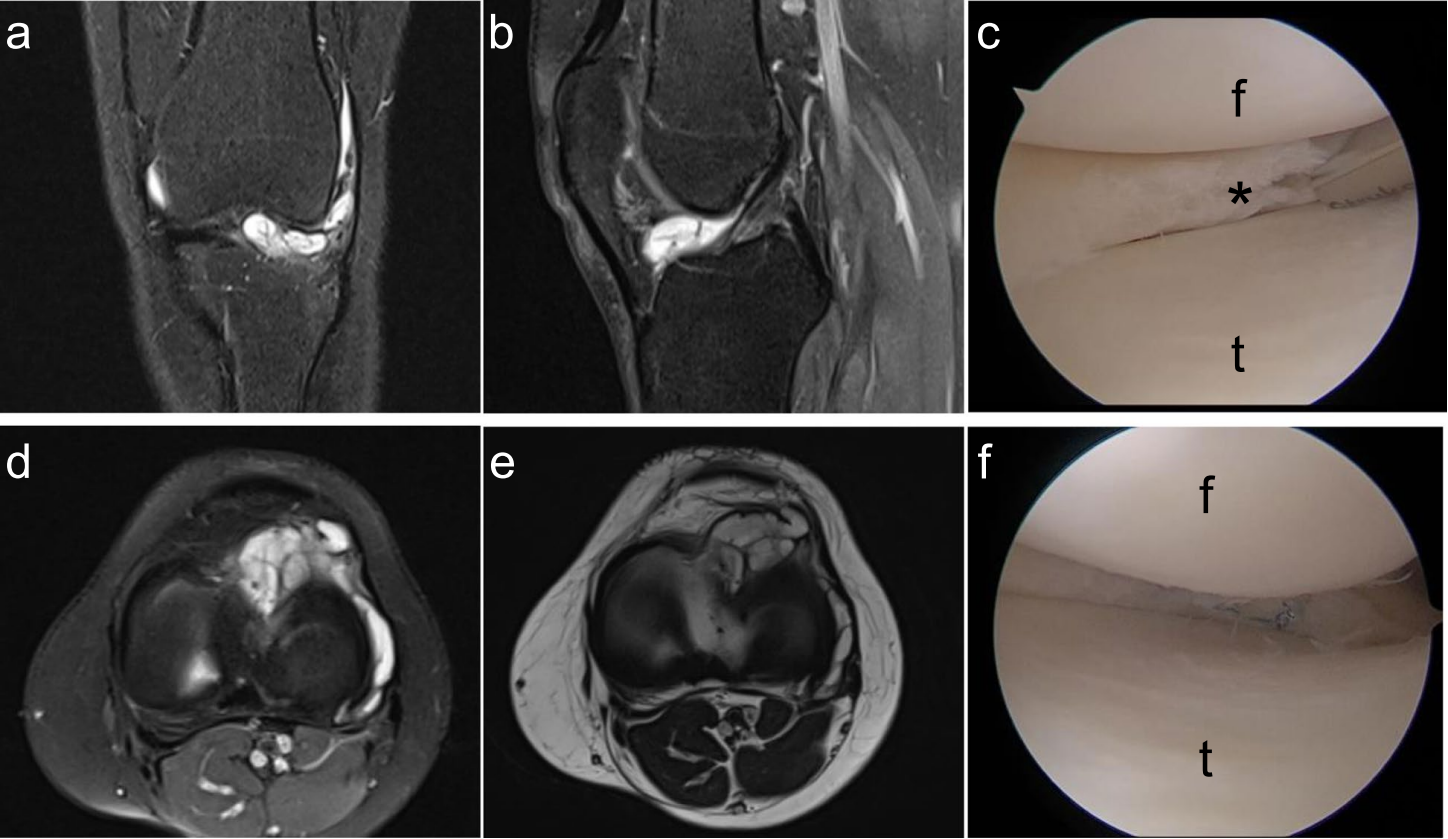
a and b Coronal and sagittal magnetic resonance image (MRI) of left knee with lateral-sided parameniscal cyst. c arthroscopic view of horizontal meniscal tear (*). d and e Axial view of MRI of the lateral-sided parameniscal cyst. f all-inside meniscal sutures. t: tibia, f: femur
